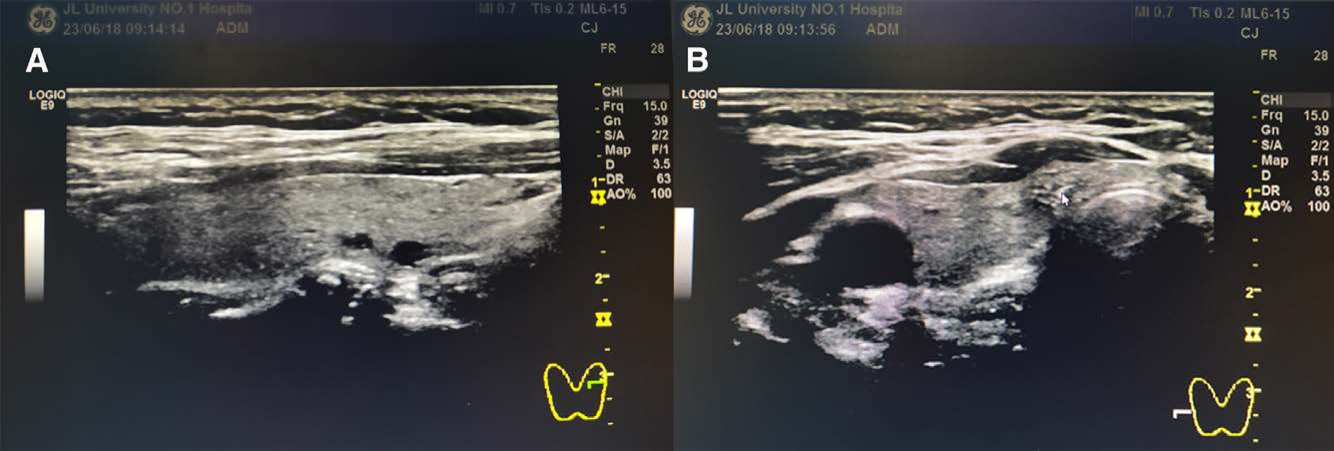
(A) Ultrasound of the left lobe of the thyroid gland showing a lower-middle mass with a regular shape and clear margins. (B) Ultrasound of the right lobe of the thyroid gland showing a lower mass with indistinct borders, irregular shape and internal calcified spots.
